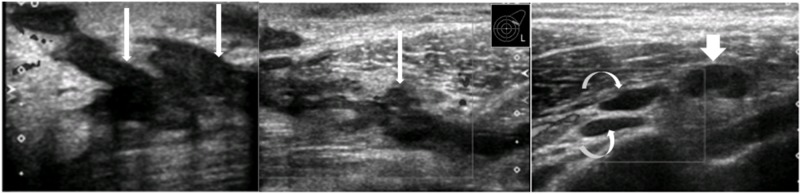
Ultrasound Irregular communicating branching sinus tracts (long white arrows) in the upper outer quadrant of left breast, extending towards axilla (curved white arrows). Enlarged left axillary lymph node (short thick white arrow).
